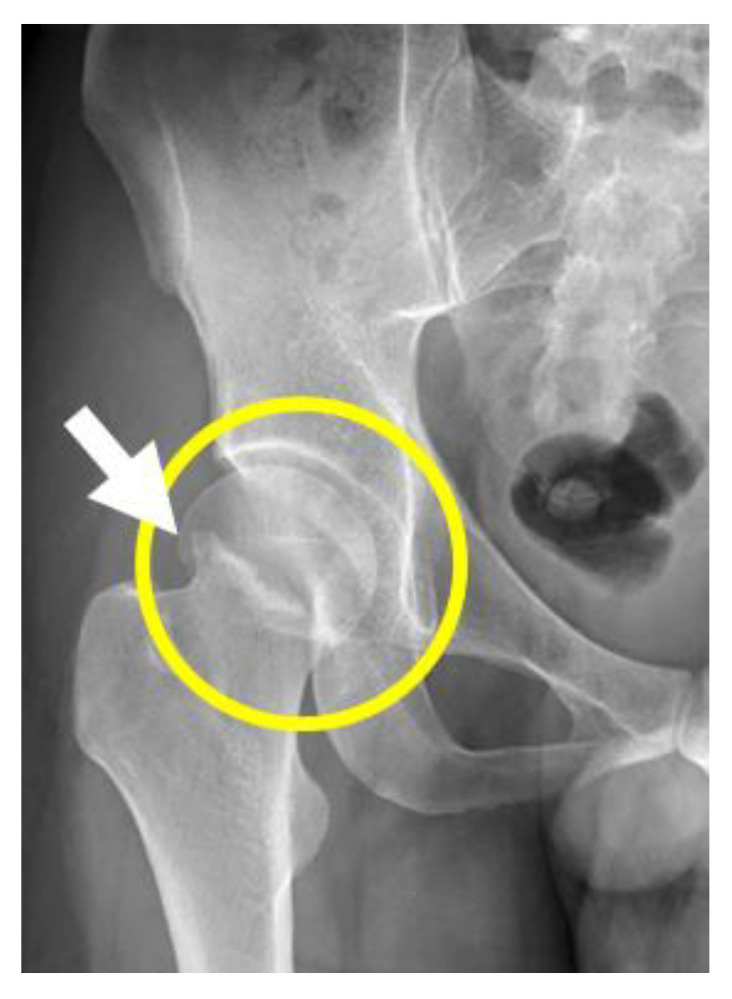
Lateral view X-ray of the right hip femoral neck fracture, Garden type I.
